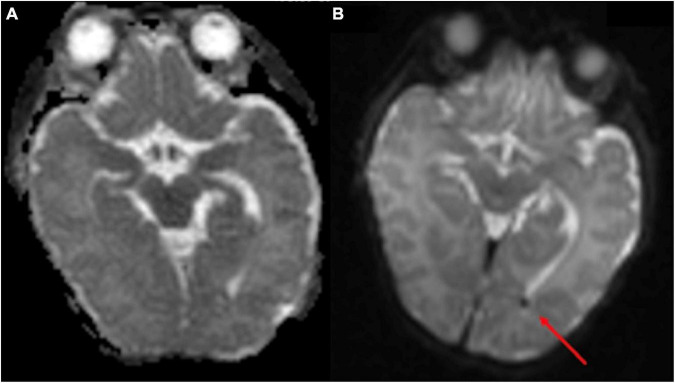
The point-of-care 1-Tesla routine pre-discharge MRI in a 4-month-old neonate was terminated after obtaining DWI/ADC due to infant movement (A). Follow-up 3-Tesla MRI 5 days later showed layering IVH in occipital horns, which could be appreciated even on the ADC series (B). No transcranial ultrasound was obtained.
